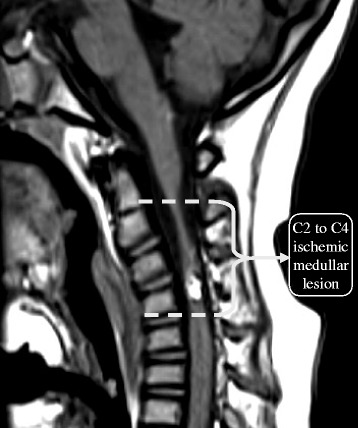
Brain MRI image (T2-weighted) performed on day 90 showing extensive cervical cord fibrosis and atrophy, which was more severe at C2–C3–C4 levels
